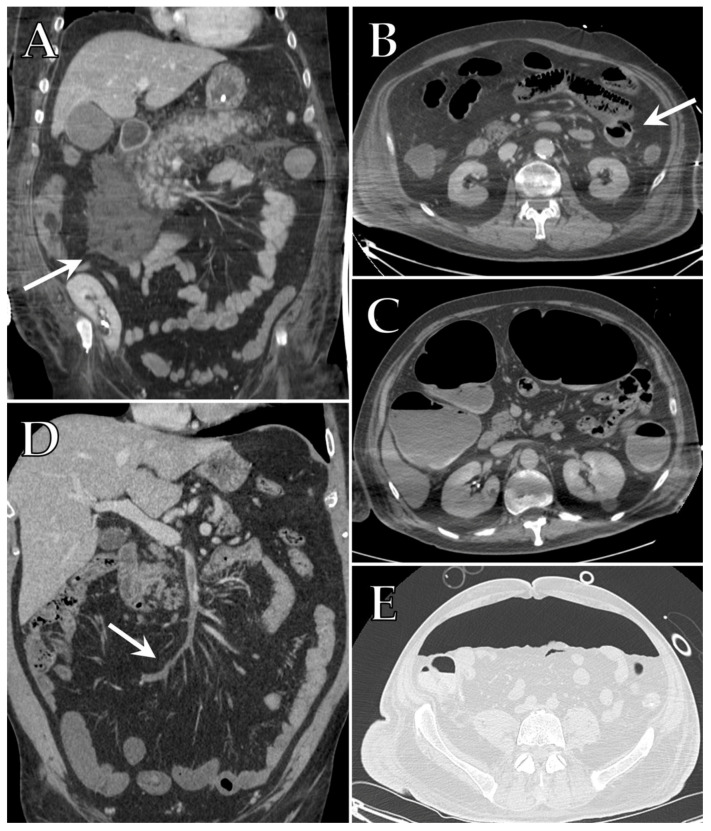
Coronal contrast-enhanced CT image of a patient with COVID-19 admitted to ICU with suspected pancreatitis reveals a diffusely enlarged pancreas, with ill-defined borders, stranding of the peri-pancreatic fat and streak of fluid along the anterior conal fascia (white arrow) (A). Axial contrast-enhanced CT scan of different COVID-19 patients with suspected acute mesenteric ischemia demonstrating the presence of linear collections of gas inside the small bowel wall referable to pneumatosis intestinalis (white arrow) (B) and dilated intestinal loops with a paper-thin wall and air-fluid levels (C). Coronal contrast-enhanced CT image of a COVID-19 patient admitted to ICU showing thrombosis of the superior mesenteric vein extending to several jejuno-ileal branches (white arrow), with consequent loss of contrast enhancement of the corresponding vascularized loops (D). Axial unenhanced CT scan with lung window of an ICU patient with COVID-19 and abdominal pain, showing a large quantity of free air (E).
